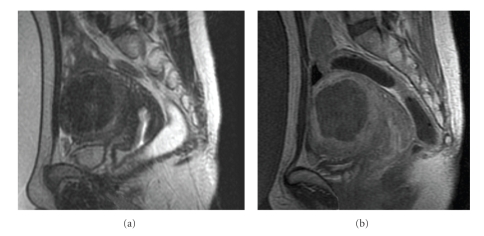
Treatment images: (a) Sagittal T2-weighted planning image, (b) Sagittal contrast-enhanced T1-weighted posttreatment image showing 90% of nonenhancing volume on the left fibroid.
